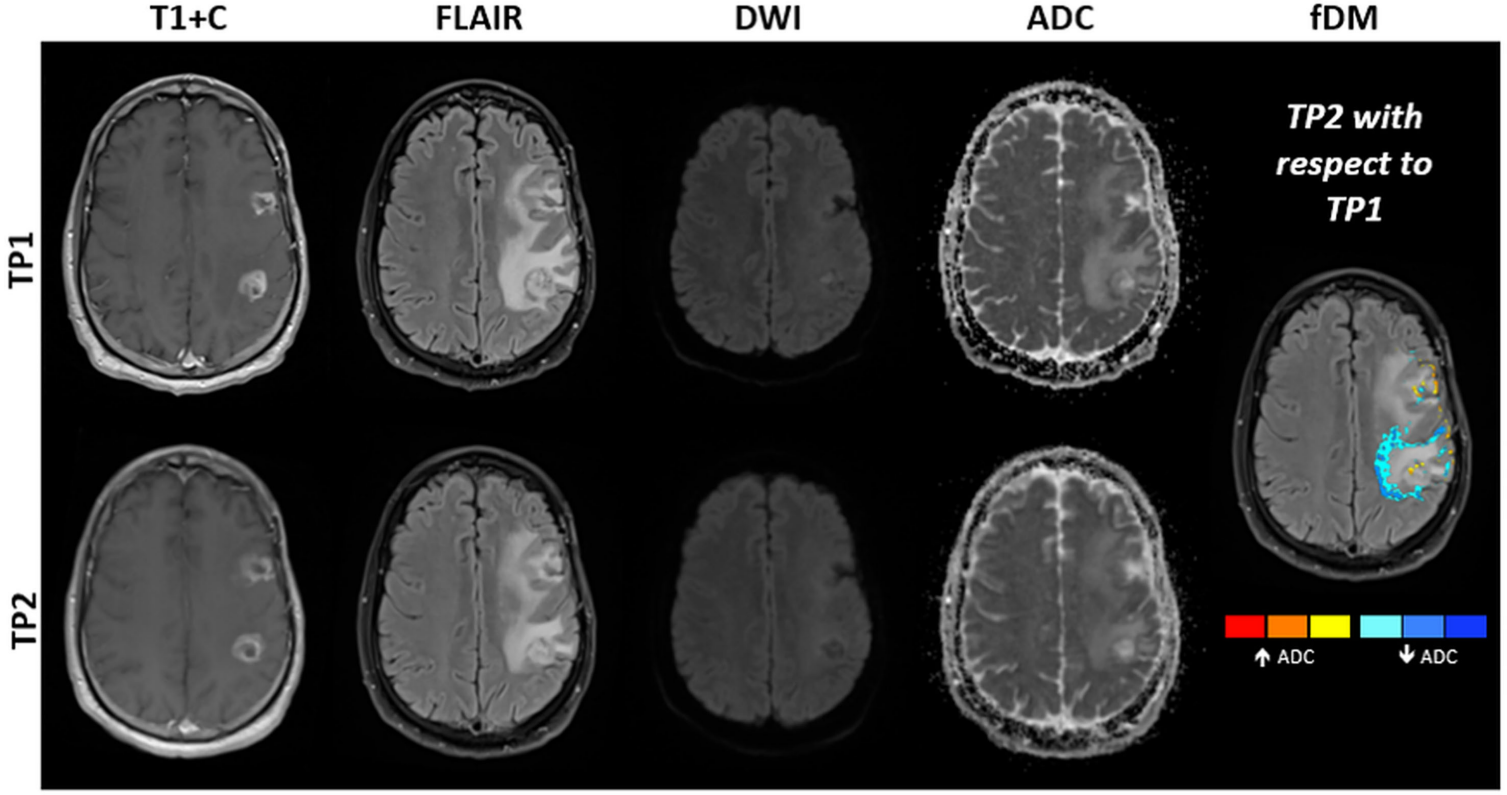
MRI obtained in a 48-year-old patient with mulitfocal glioblastoma, IDH-wildtype. Shown are post-contrast *T*_1_-weighted images, FLAIR images, DWI at b = 1000 s/mm^2^ and ADC maps obtained at approximately two (TP1) and three months (TP2) after standard-of-care radiotherapy and concomittant temozolomide. Subtraction of ADC obtained at TP1 from ADC obtained at TP2 results in the creation of fDMs, which show where ADC is increasing (red to yellow) or decreasing (blue). Decreasing ADC (blue regions) is suggestive of increasing tumor cell density. Surgical resection performed one week after TP2 revealed an admixture of tumor recurrence and post-treatment related effects. Note that interpretation can be complicated by resolving edema between time points, which may also show decreased ADC.
